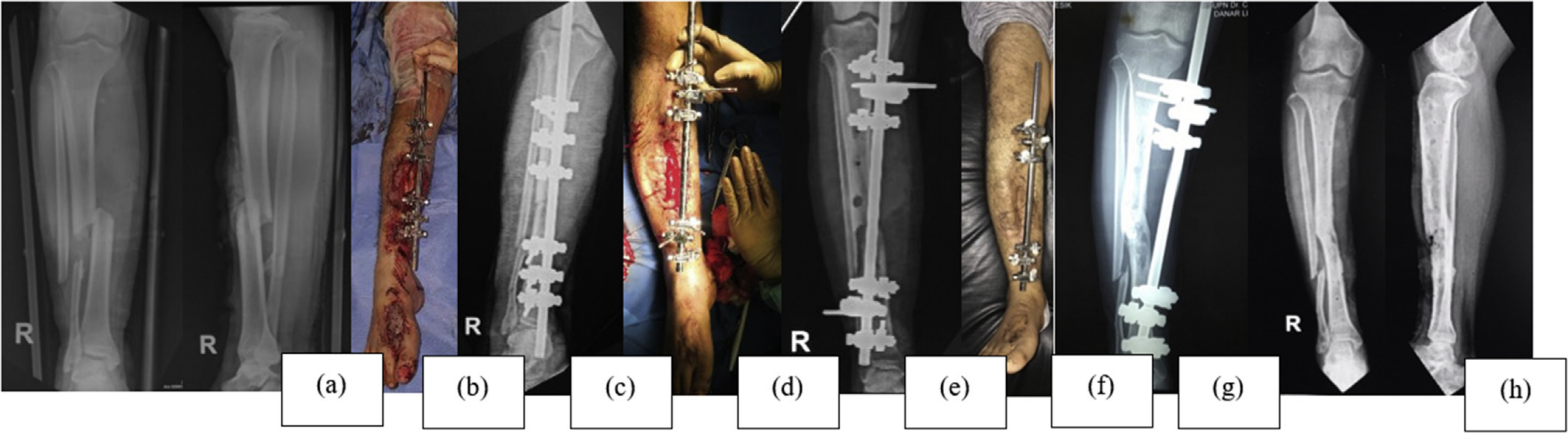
Case 2; a 24-year-old male with open fracture of the right tibial shaft. (a) Initial anteroposterior and lateral X-ray of the tibia. (b) Anterior preoperative view of the right leg. (c) Preoperative anteroposterior X-ray of the right leg. (d) Anterior postoperative view of the right leg – revision of external fixation more proximal and sliced cancellous bone graft. (e) Postoperative anteroposterior X-ray of the right leg. (f) Anterior view of the right leg, 13 months postoperatively – healed wound. (g) Anteroposterior X-ray of the right leg, 13 months postoperatively – bony union. (h) Post-implant removal
